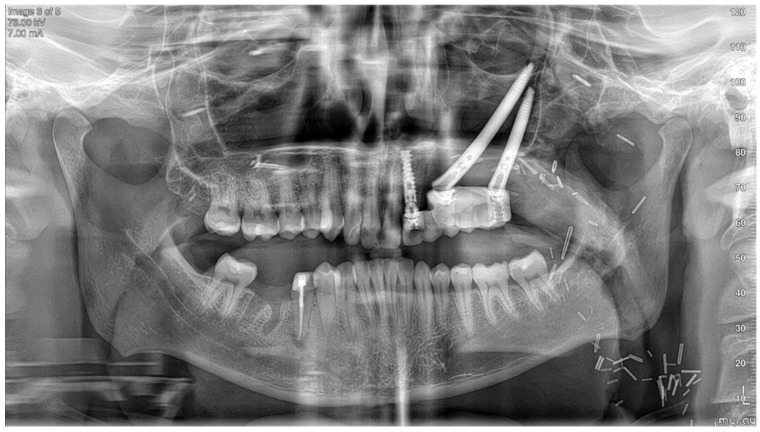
Postoperative panoramic X-ray.
